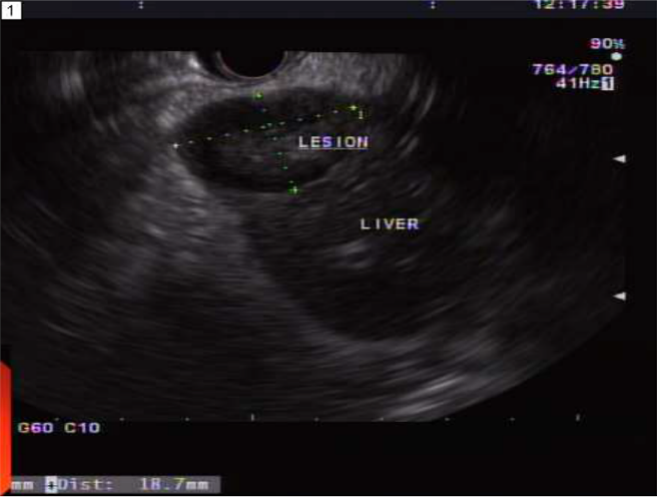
Ultrasound appearance of hepatic lesions.
